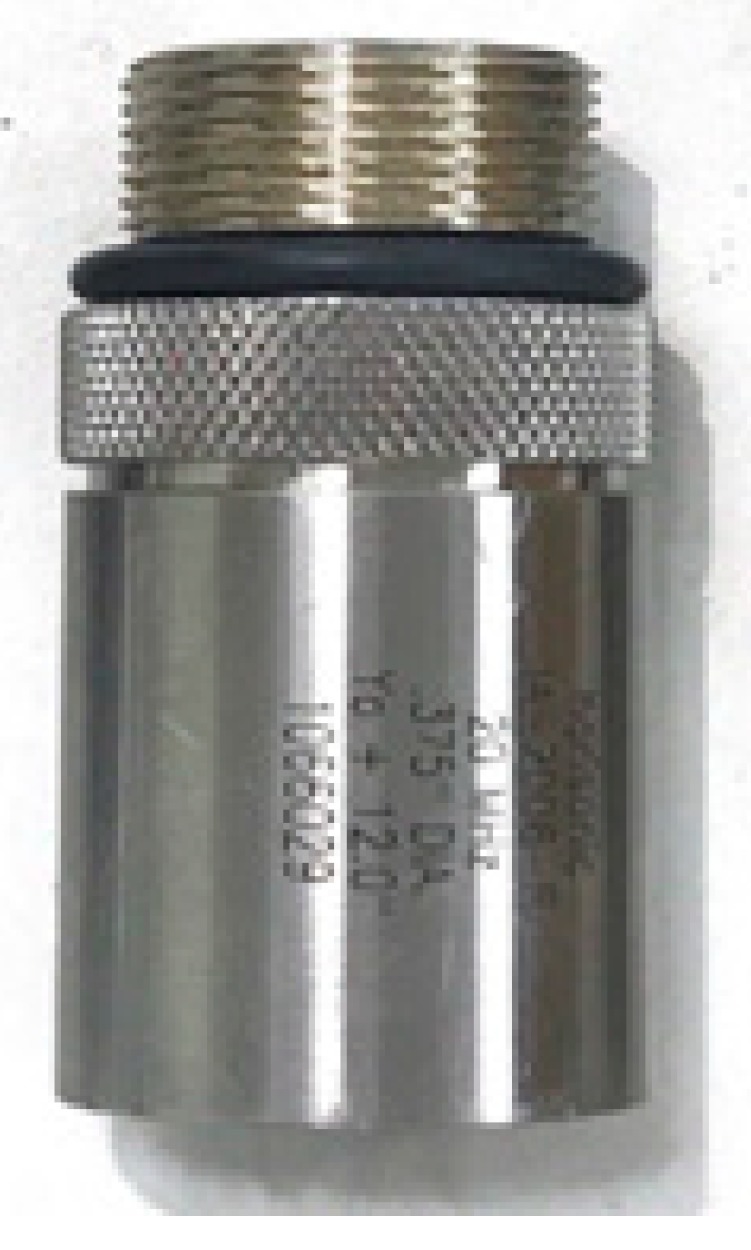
Twenty megahertz ultrasound transducer.
